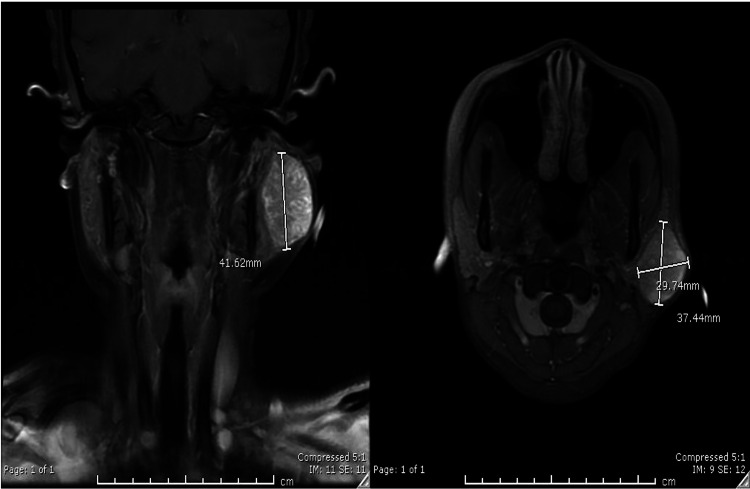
MRI orbit/face/neck without contrast which revealed an indeterminate complex heterogeneous enhancing mass within the superficial left parotid gland.
